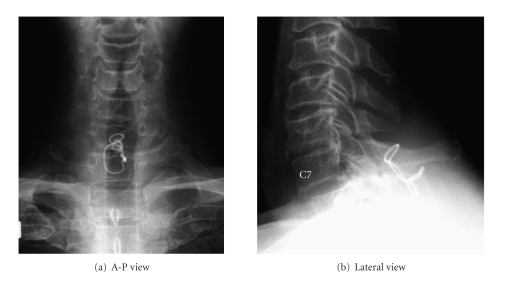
Radiographs one year postoperatively.
